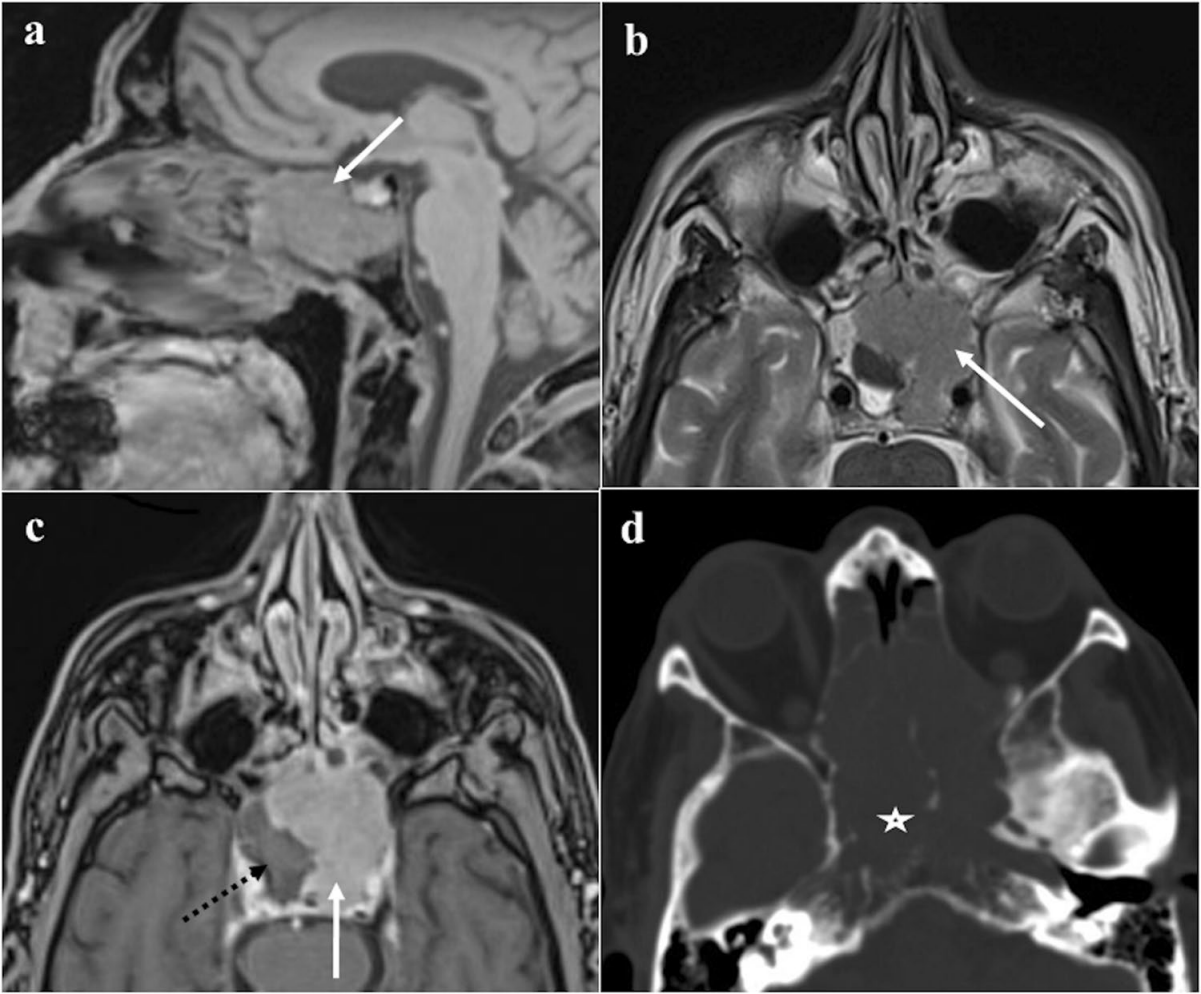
Primary lymphoblastic lymphoma of the left sphenoid sinus in a 79-year-male patient with headache. MRI reveals a homogeneous soft tissue mass in the left sphenoid sinus (white arrows) showing intermediate SI on sagittal T1W (a) and axial T2W (b) images and moderate homogeneous enhancement after intravenous gadolinium contrast agent on axial T1W fat-saturated images (c). The right sphenoid sinus is filled by partially dehydrated mucus due to the drainage obstruction (c, black dotted arrow). Bone algorithm reconstruction CT obtained one month later (d) shows a rapid growth of the lesion with massive destruction of the clivus (*)
